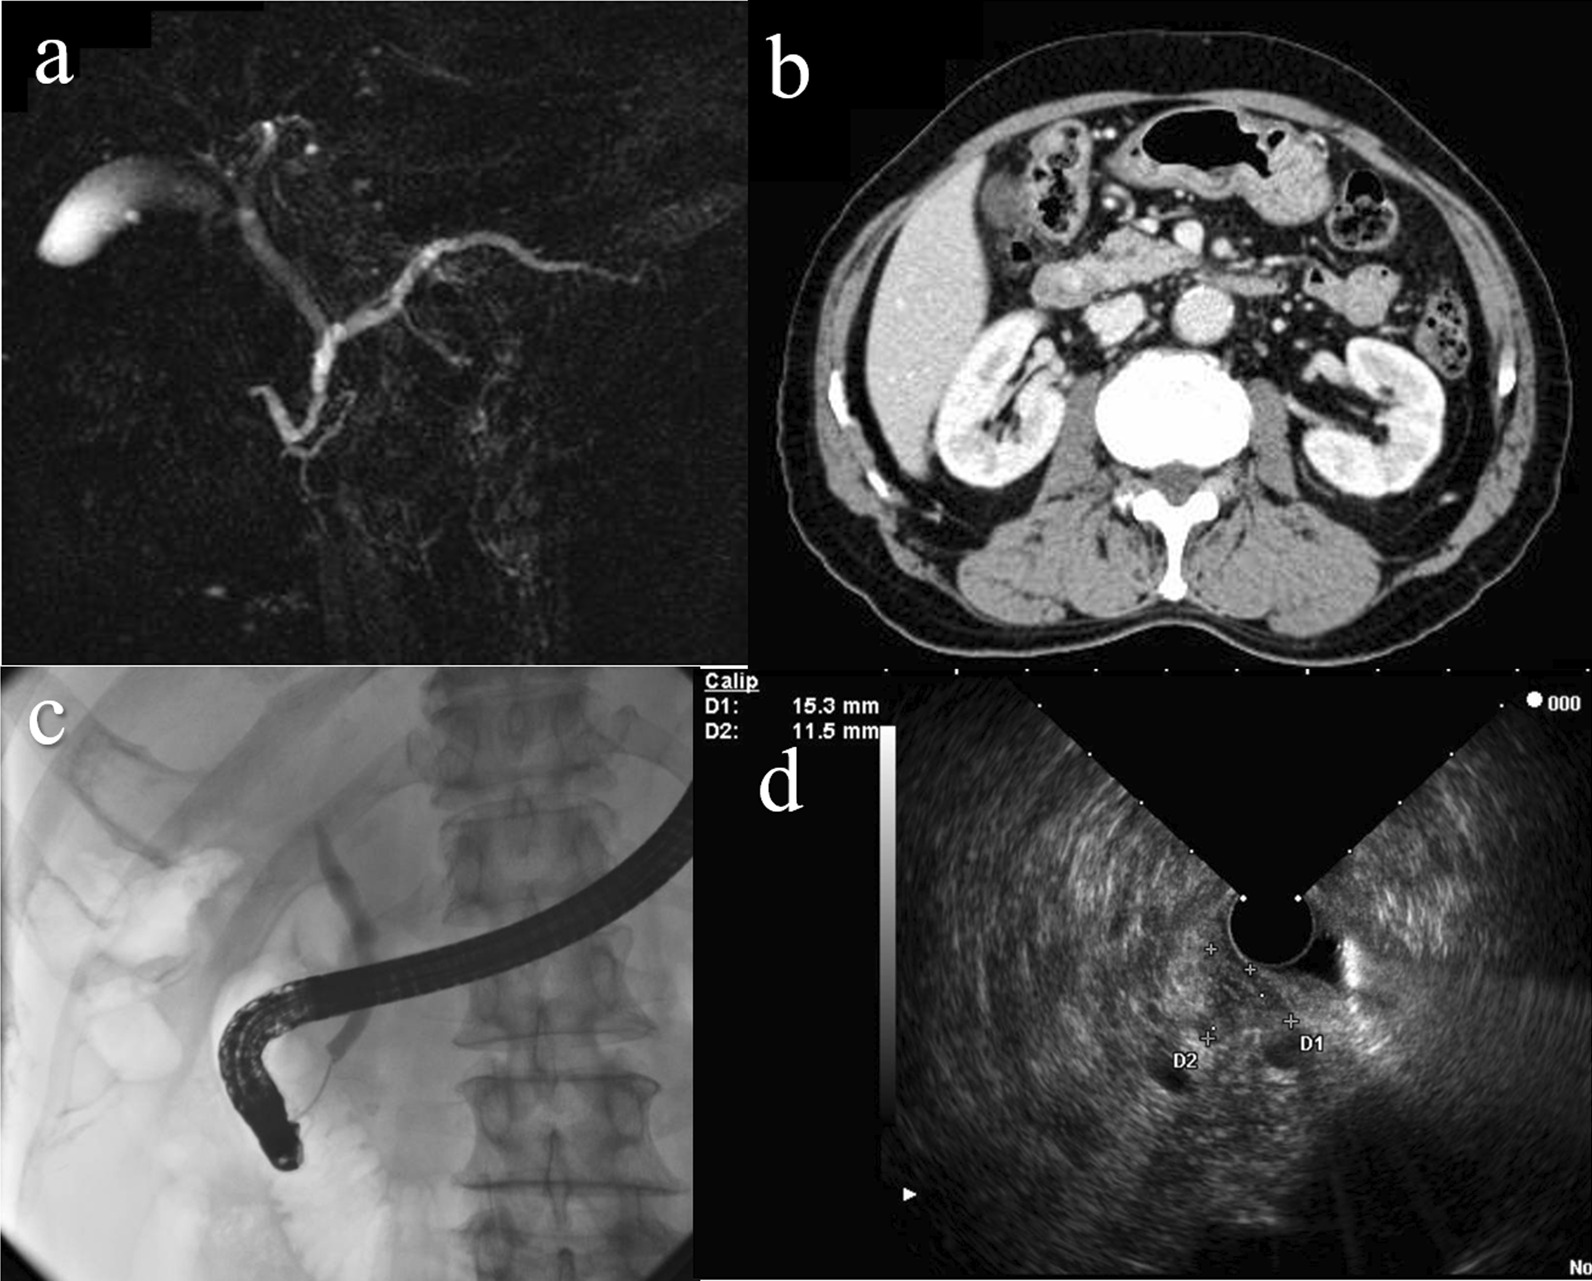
a Magnetic resonance cholangiopancreatography showing a dilated dorsal pancreatic duct that was not connected with the ventral pancreatic duct and opened to the minor papilla. The common bile duct had no communication with the pancreatic main duct and opened to the ampulla of Vater. b Contrast-enhanced computed tomography scan showing a 12-mm hypervascular mass near the ampulla of Vater and the dilated main pancreatic duct. c Injection of contrast through the minor papilla revealing the dilated dorsal pancreatic duct. d Endoscopic ultrasonography showing a defined hypoechoic mass in the minor papilla with no invasion
